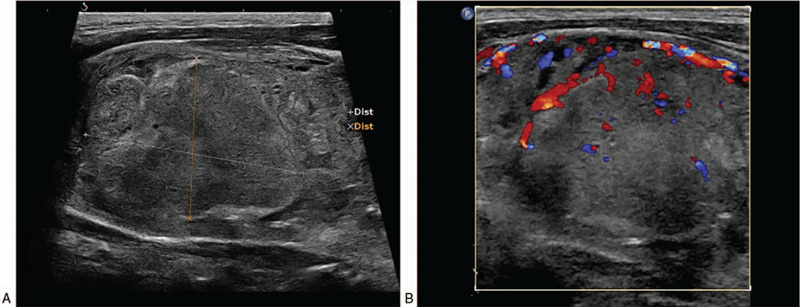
Ultrasound images of the patient in 2018. (A) A 2-dimensional image showing the size of the relatively big nodule of about 5.6 × 3.7 cm in the right lobe. (B)CDFI shows rich blood flow signals around and inside the nodule.
